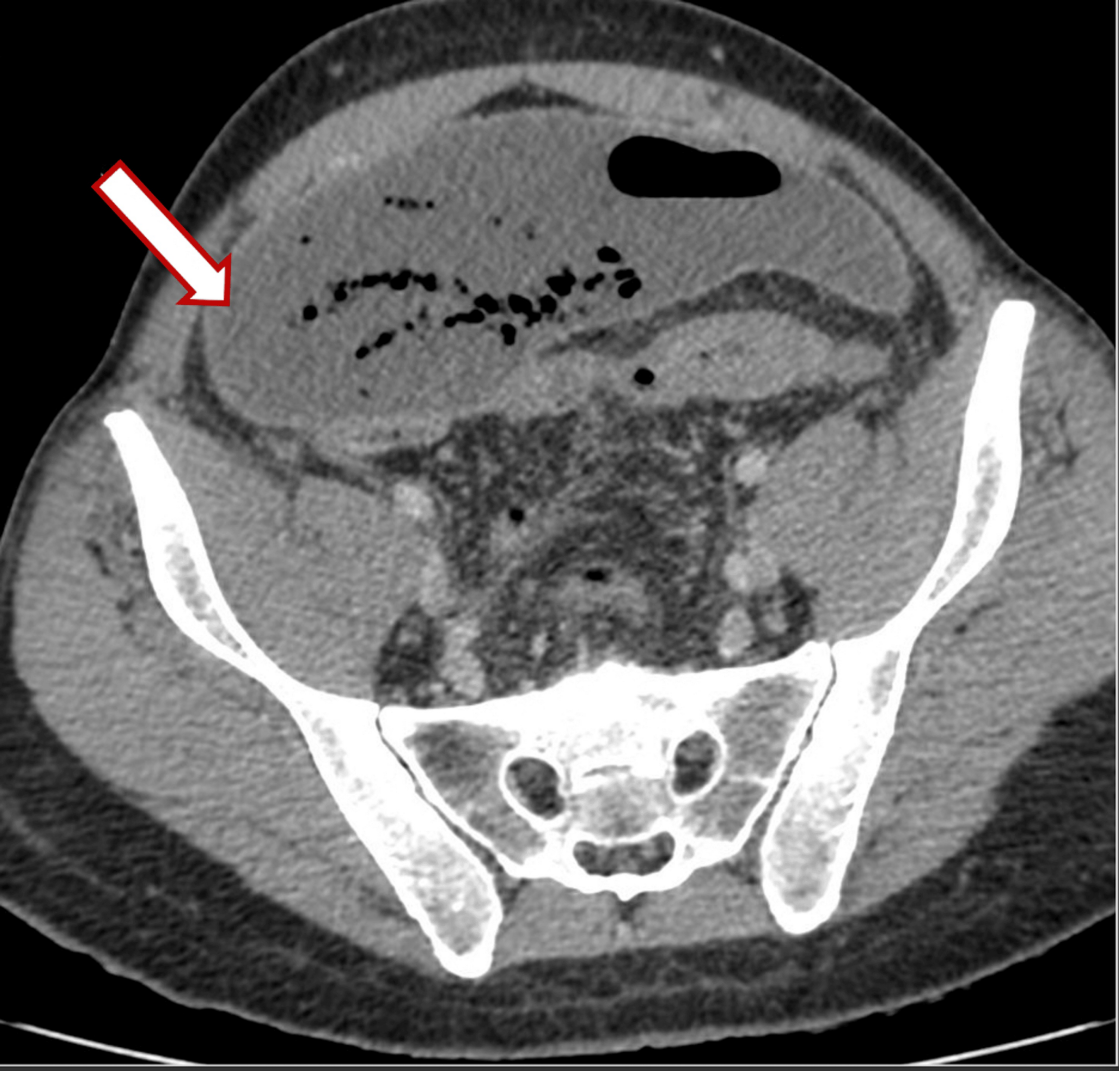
CT imaging demonstrating a large abscess cavity in the lower abdomen containing air and fluid, extending anteriorly to the ventral abdominal wall, with maximal dimensions of 20 cm (TS) × 8 cm (AP) × 19 cm (CC). The collection contacts the distal sigmoid colon, with a few tiny adjacent air locules indicating the site of perforation. The white arrow (outlined in red) indicates the abscess cavity. TS: transverse; AP: anteroposterior; CC: craniocaudal
